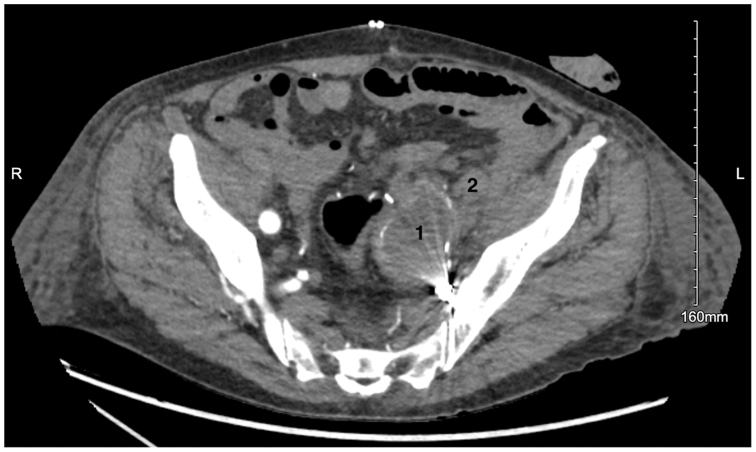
Postoperative computed tomography (CT) axial cut of the pelvis 11 days postoperatively showing the ligated left internal iliac artery (*1*) as well as the ligated left external iliac artery (*2*).
